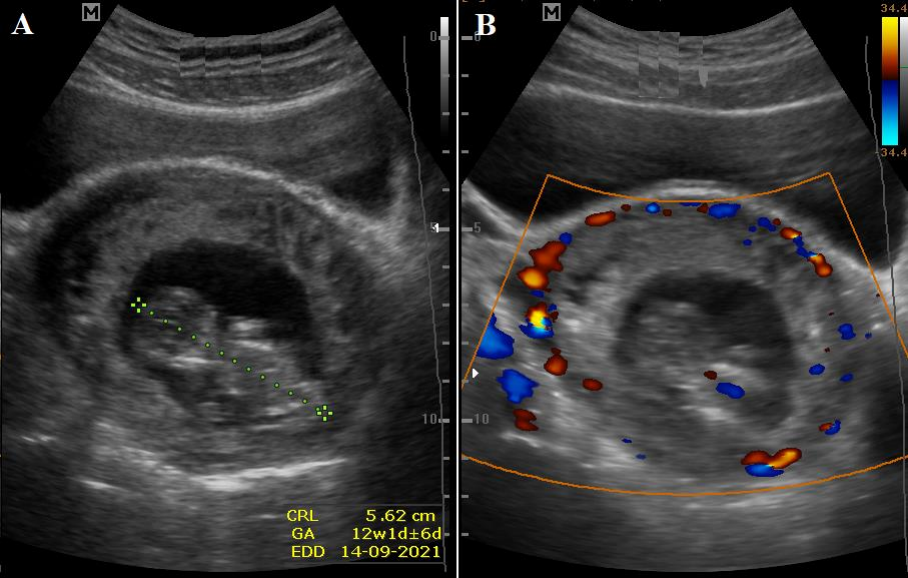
two transabdominal ultrasound images showing live cervical ectopic gestation: A) fetal pole with a Crown-rump length of 5.62 cm and a gestational age of 12 weeks+1 day; B) mass completely invaded both cervical lips and shows vascularity under Doppler color interrogation, fetal heart vascularity is seen
